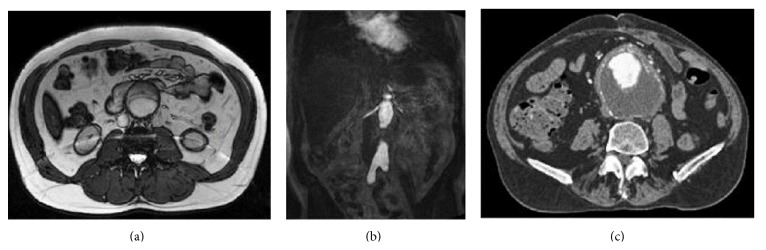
(a) MR transversal bSSFP for thrombus and outer wall segmentation; (b) MR coronal FLASH for lumen segmentation; (c) CTA image for shape modelling.
